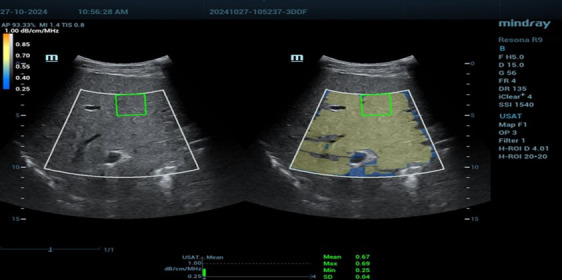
Asymptomatic 25 yr old male patient who is addict on alcohol (urine test +Ve) for 5 years with elevated liver enzymes. Liver attenuation value obtained by two dimensional shear wave elastography technique (USAT technique by resona R9 ultrasound machine of mindray medical systems) with the mean was 0.67 dB/cm/MHz indicating grade 1 liver steatosis with respect to manufacturer reliability and stability index and measurement IQR/median <10. With standardized box positioning.
